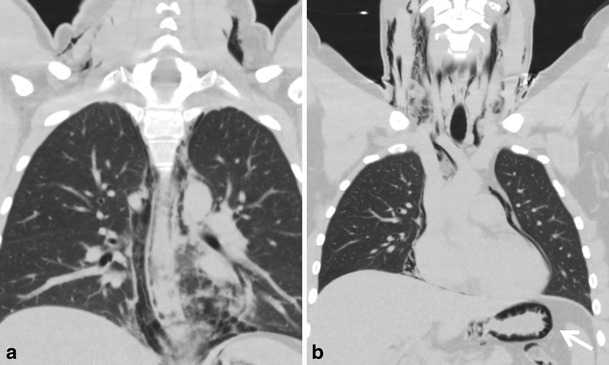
A 25-year-old male presented at the ED with complaints of chest pain, radiating to the shoulders. The pain started during evening dinner with an impacted food bolus. His history revealed dysphagia. At the ED he acutely developed dyspnoea after drinking water. Subcutaneous emphysema developed. A routine chest X-ray and thoracic CT examination were performed for further evaluation. (a) The coronal thoracic CT shows intramural gas extending from the level of the upper oesophagus to the (b) stomach (arrow) accompanied with extensive pneumopericardium, pneumomediastinum, and subcutaneous emphysema, due to perforated oesophageal dissection. At endoscopy, two foci of perforation in the distal oesophagus were found, which were successfully treated by placement of a (retrievable) covered stent
